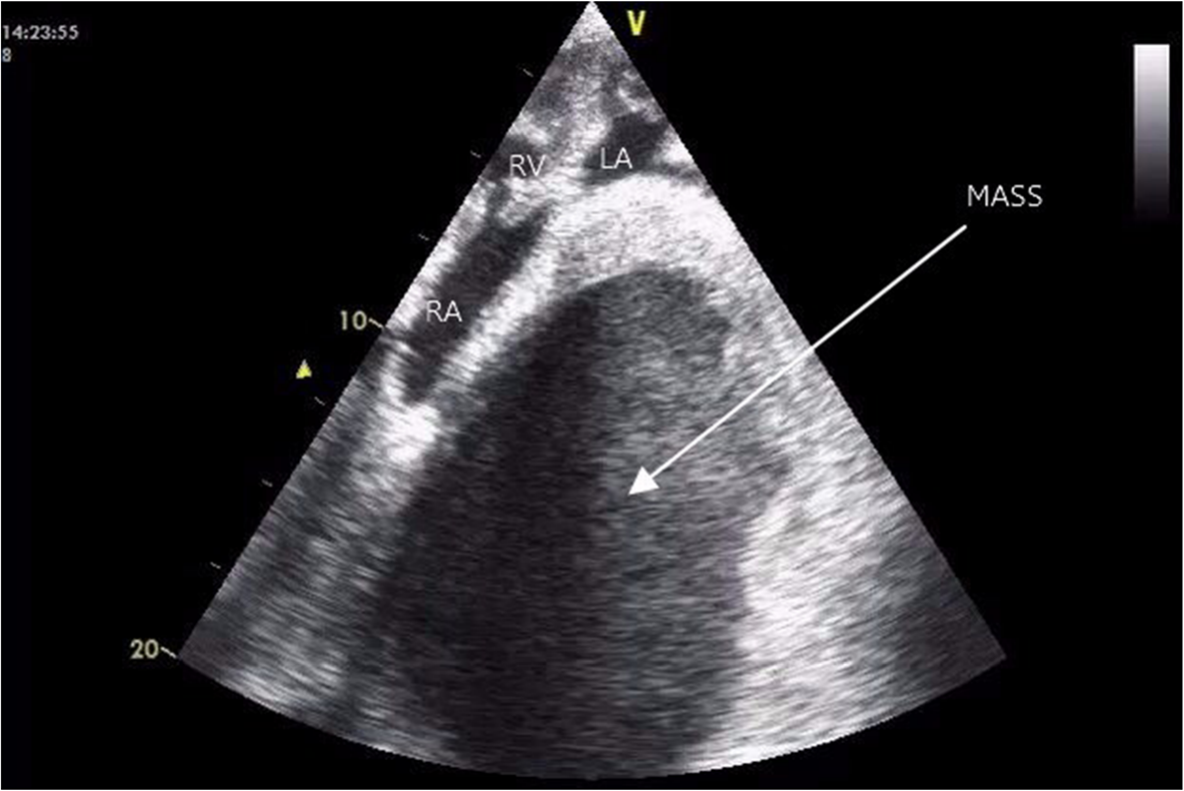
Echocardiogram (apical four-chamber view) displaying a huge mass (4.6×3.3 cm) with spontaneous ECHO contrast (arrow) compressing the heart inferiorly
